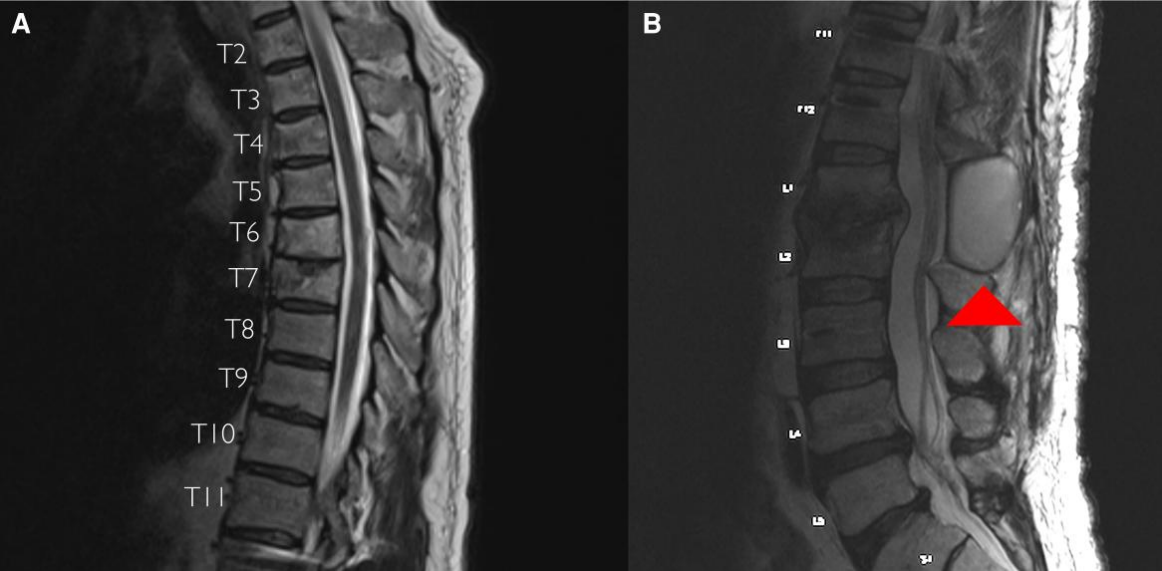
At month 9 of therapy, T2-weighted magnetic resonance imaging of the thoracic spine (*A*) and lumbar spine (*B*) in sagittal views. The arrowhead highlights evolving purulence at the lumbar laminectomy site, in the background of otherwise improving tuberculous infection.
